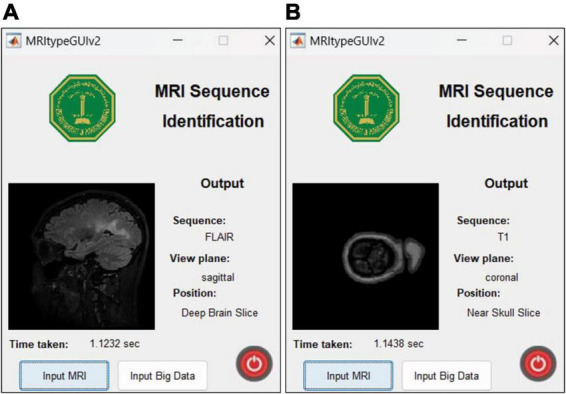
MRI Sequence Identification UI. Correct classification of (A) deep brain FLAIR axial slice (B) near skull T1 coronal slice.
